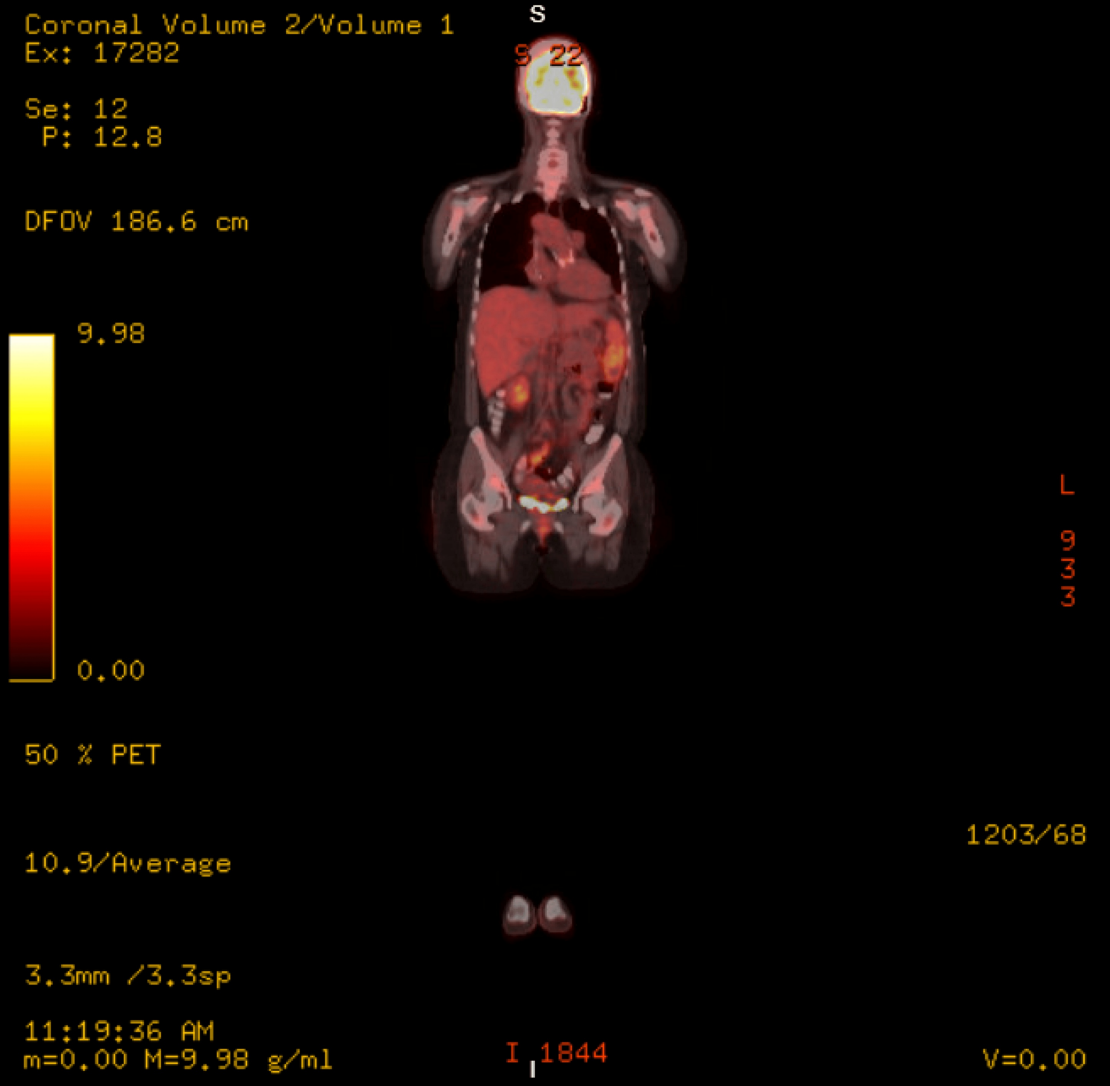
Positron emission tomography-computed tomography demonstrating a focus of fluorodeoxyglucose (FDG) uptake at the pancreatic tail associated with hypoattenuation of the parenchyma and intense focal FDG uptake at the splenic hilum in the region of the splenic vein.
